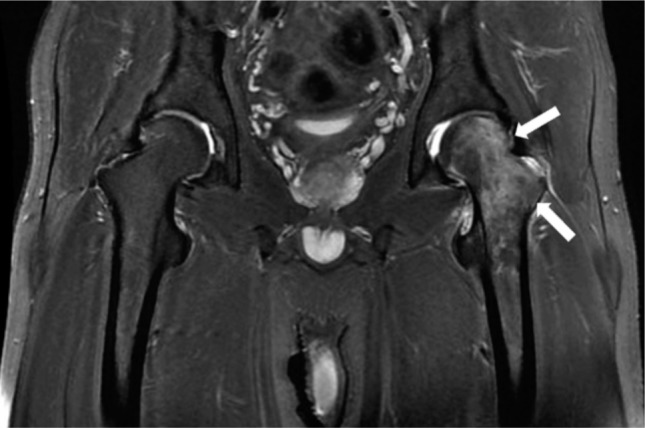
T2-weighted MRI at the second onset left hip (8 month after first onset in right hip) showing high signal intensity on left femoral neck and head (white arrows) with normal signal intensity on right side.
